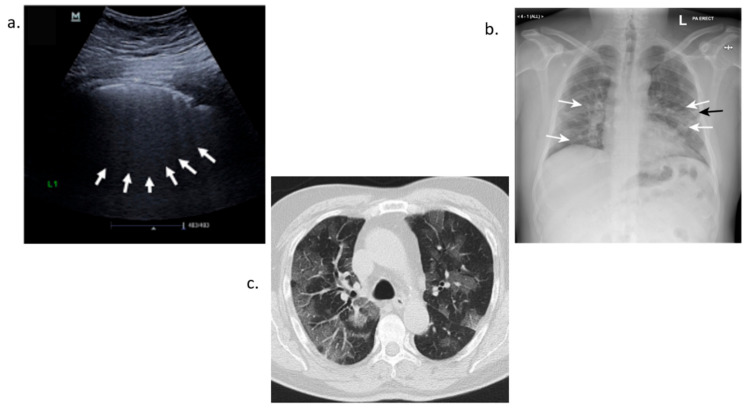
COVID-19 imaging detection (a). Ultrasound detection (b). X-ray detection (c). Computerized tomography scan.
